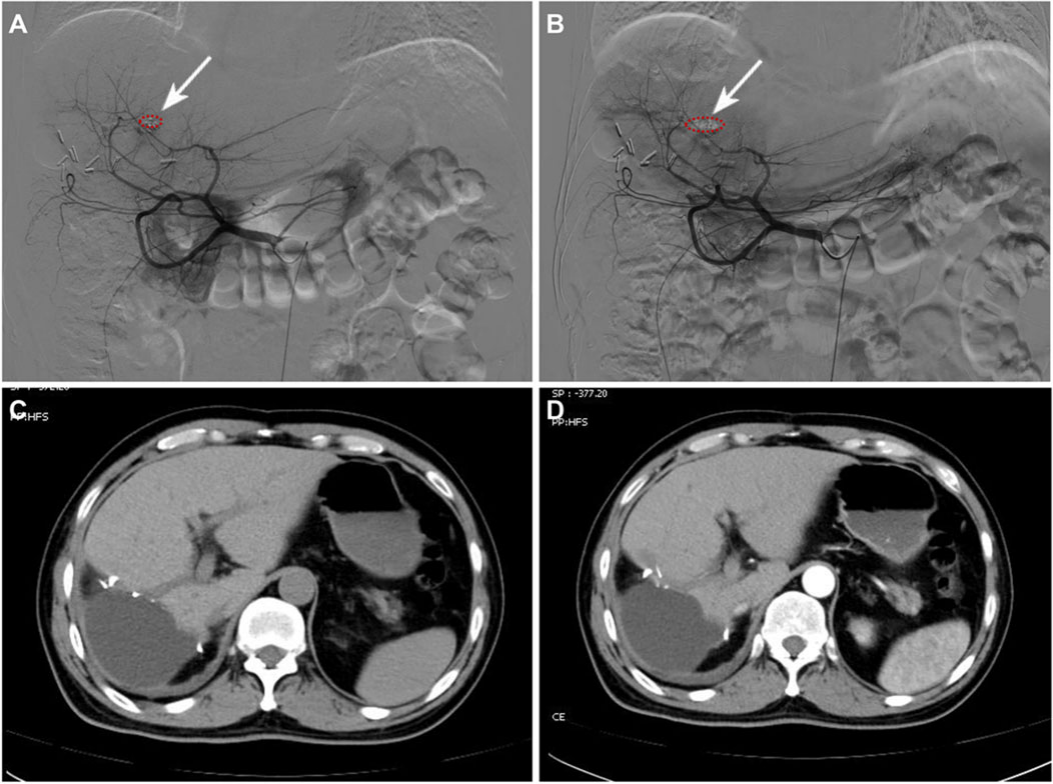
Follow-up treatment and re-examination. (A) One week after hepatectomy, the patient received the angiographic imaging. (B) The angiographic images of the patient with the treatment of infusion chemotherapy and TACE. The contrast-enhanced CT of the patient after hepatectomy three months: (C) venous phase and (D) arterial phase.
